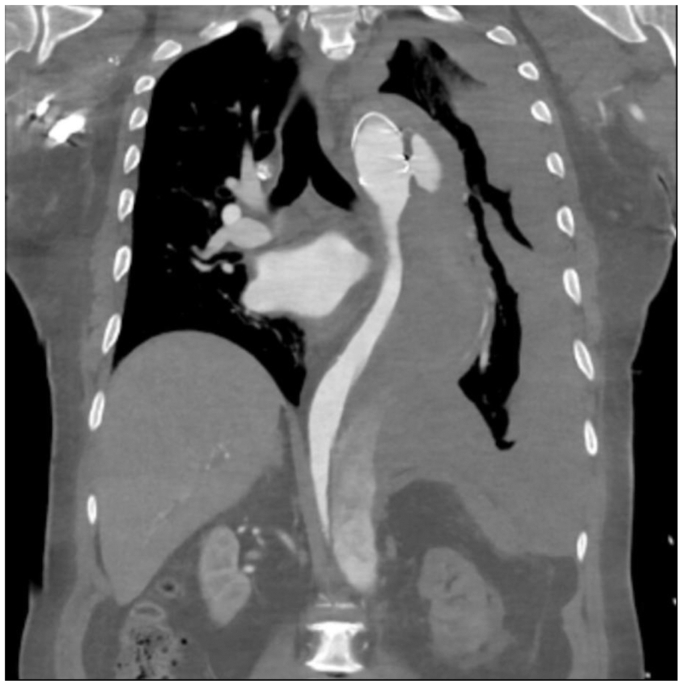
Computed tomography angiography (CTA) on presentation. A coronal image demonstrating a large intramural hematoma in the descending thoracic aorta associated with a left hemothorax.
